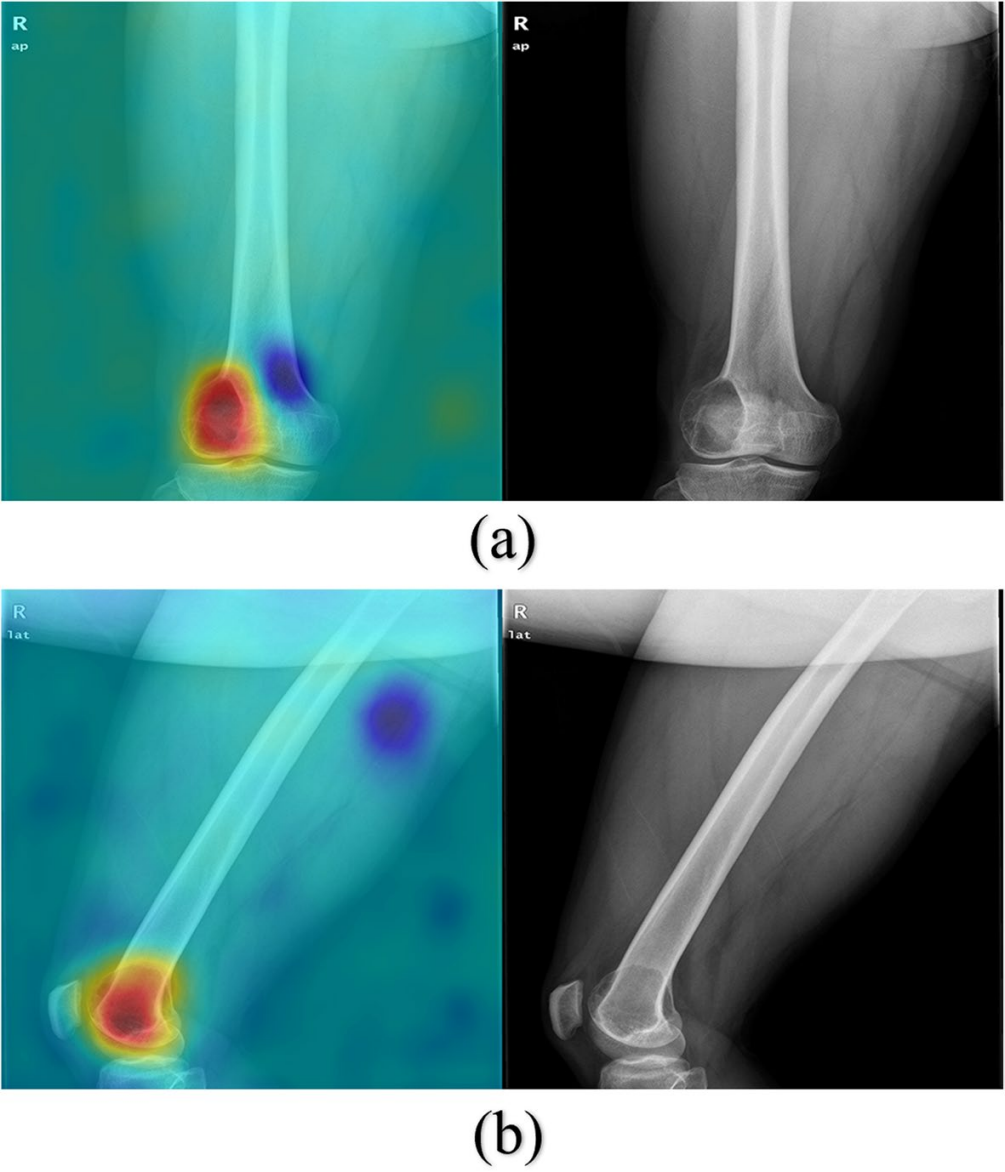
Anteroposterior (a) and lateral (b) radiographs of GCT of the distal femur and their overlapping Grad-CAM maps
